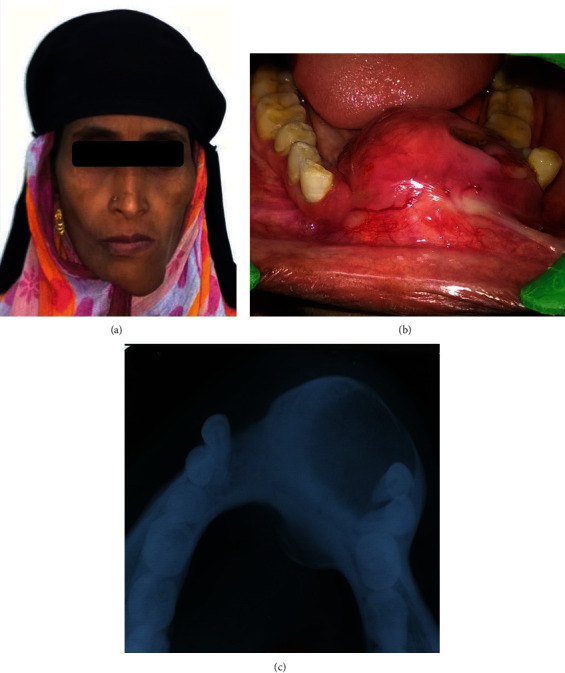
(a) Extraoral swelling; (b) intraoral ulcerated mass with obliteration of labial vestibule; (c) radiographic presentation showing the expansion of cortical plates.
